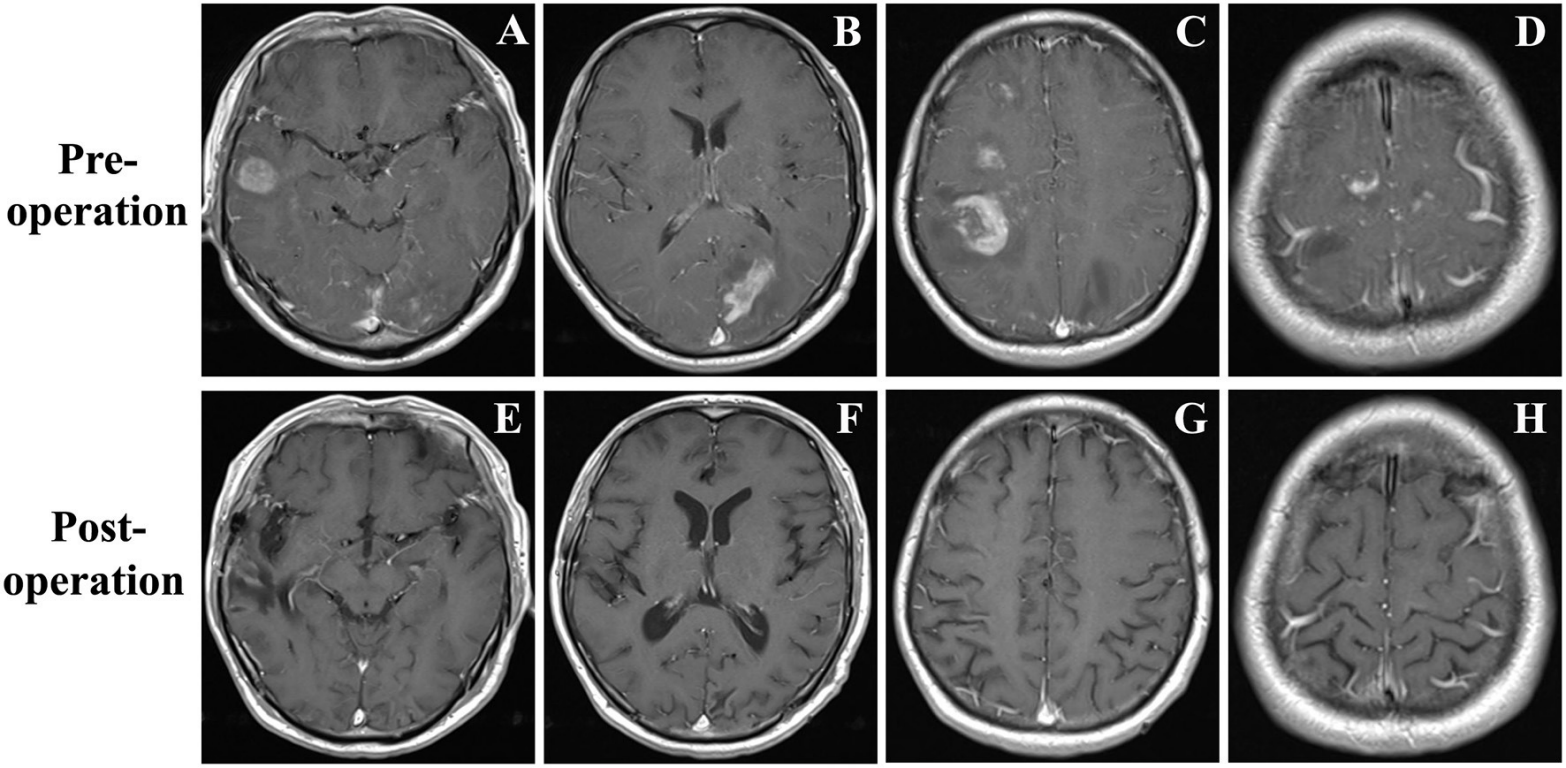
(A–D) Brain MRI demonstrated a few contrast-enhancing, cotton-like lesions up to 25 mm in diameter located in the right temporal, frontal, and parietal lobes, and left occipital lobe with perifocal edema. (E–H) Brain MRI revealed all the lesions disappeared at the fifth month of follow-up.
